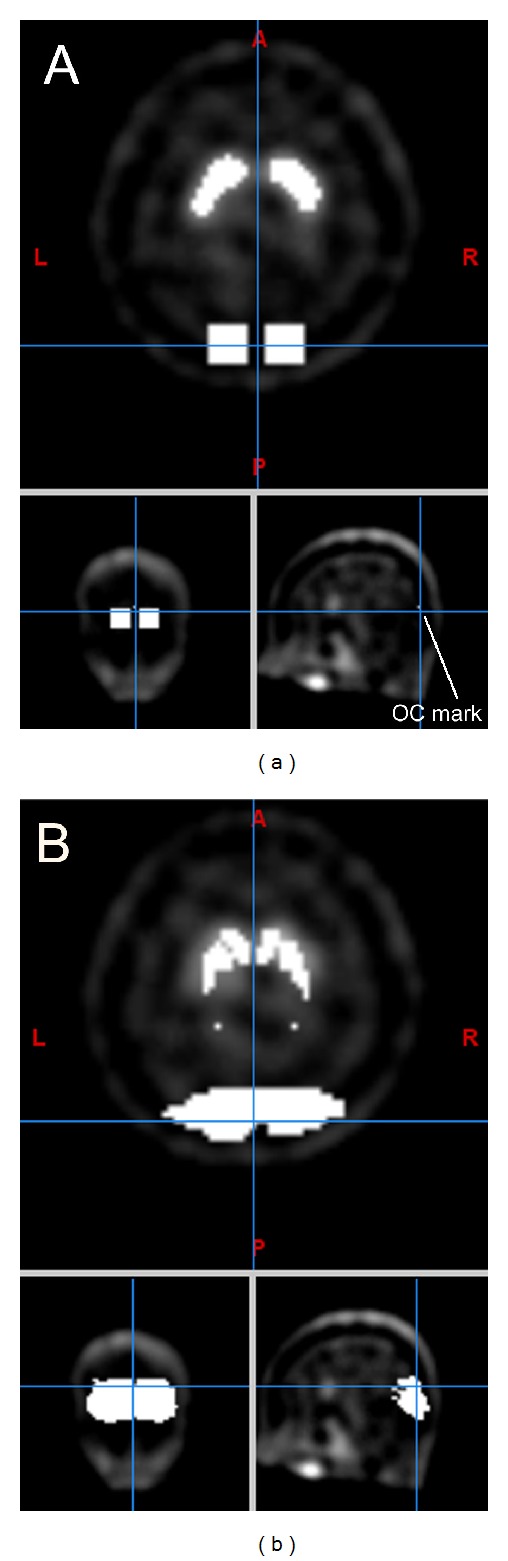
ROIs of striatum and occipital lobe in the TRODAT-1 SPECT of a 23-year-old woman (native space). (a) 70% threshold-ROI method and (b) SPECT-normalization approach.
